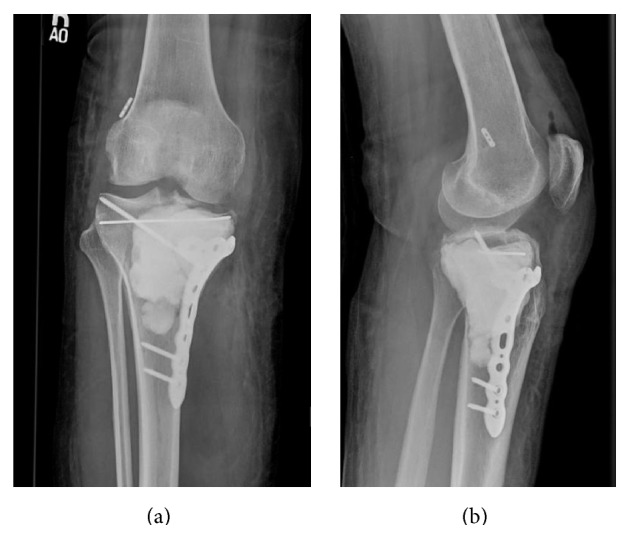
AP and lateral view radiographs immediately after curettage and packing of the proximal tibial giant cell tumor along with medial collateral ligament (MCL) repair; prophylactic fixation of the right tibia.
